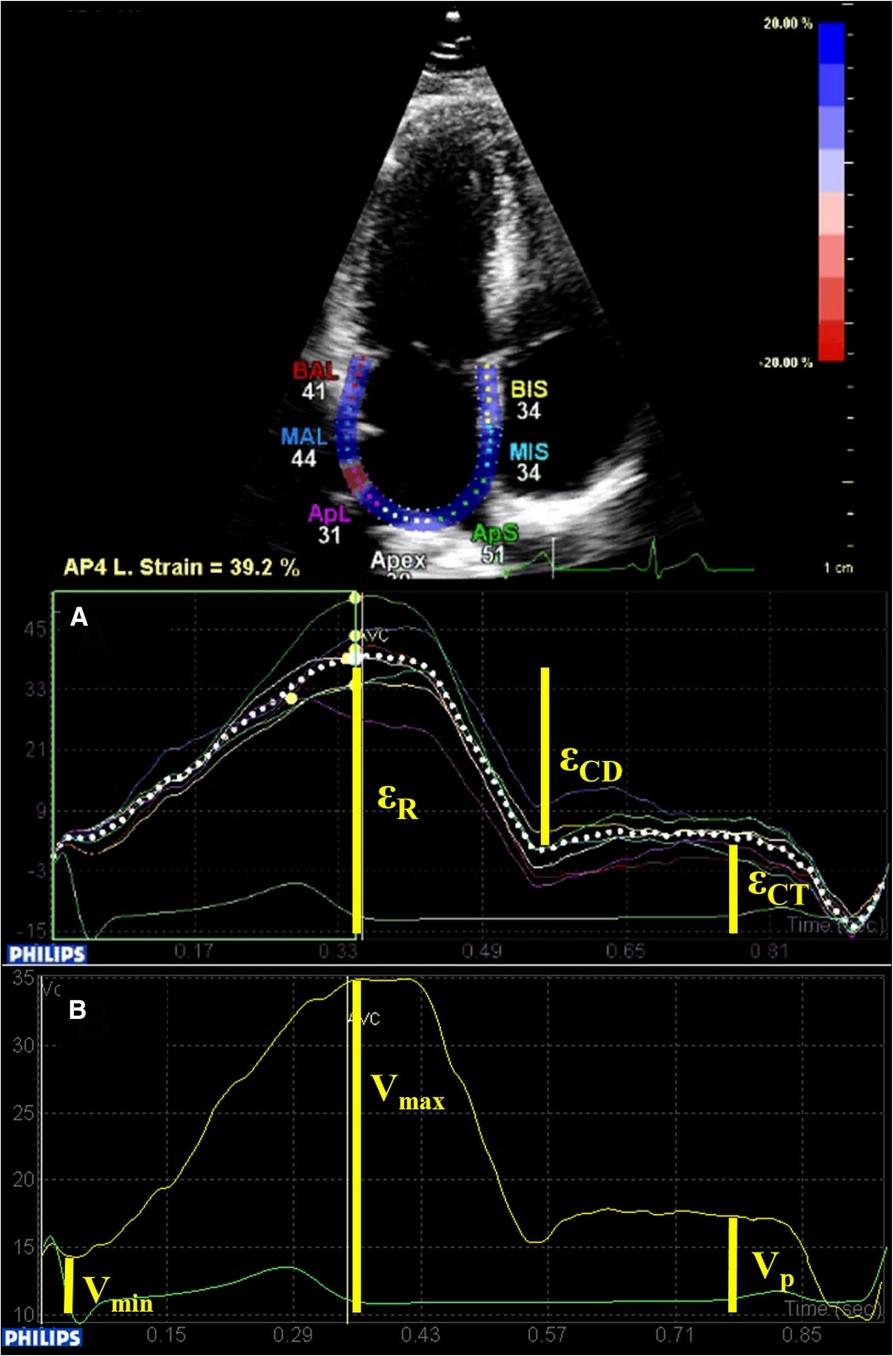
Four-chamber view image depicting the analysis of LA strain using speckle tracking technique. The region of interest is optimized manually, and then LA strain curve is created by the speckle-tracking software (a). Using the atrial borders created for speckle tracking analysis, LA volume curves are generated by the same software (b). *ε*_*R*_ Reservoir strain, *ε*_*CD*_ conduit strain, *ε*_*CT*_ contractile strain, *Vmax* maximal volume, *Vmin* minimal volume, *Vp* volume at the beginning of P wave)
